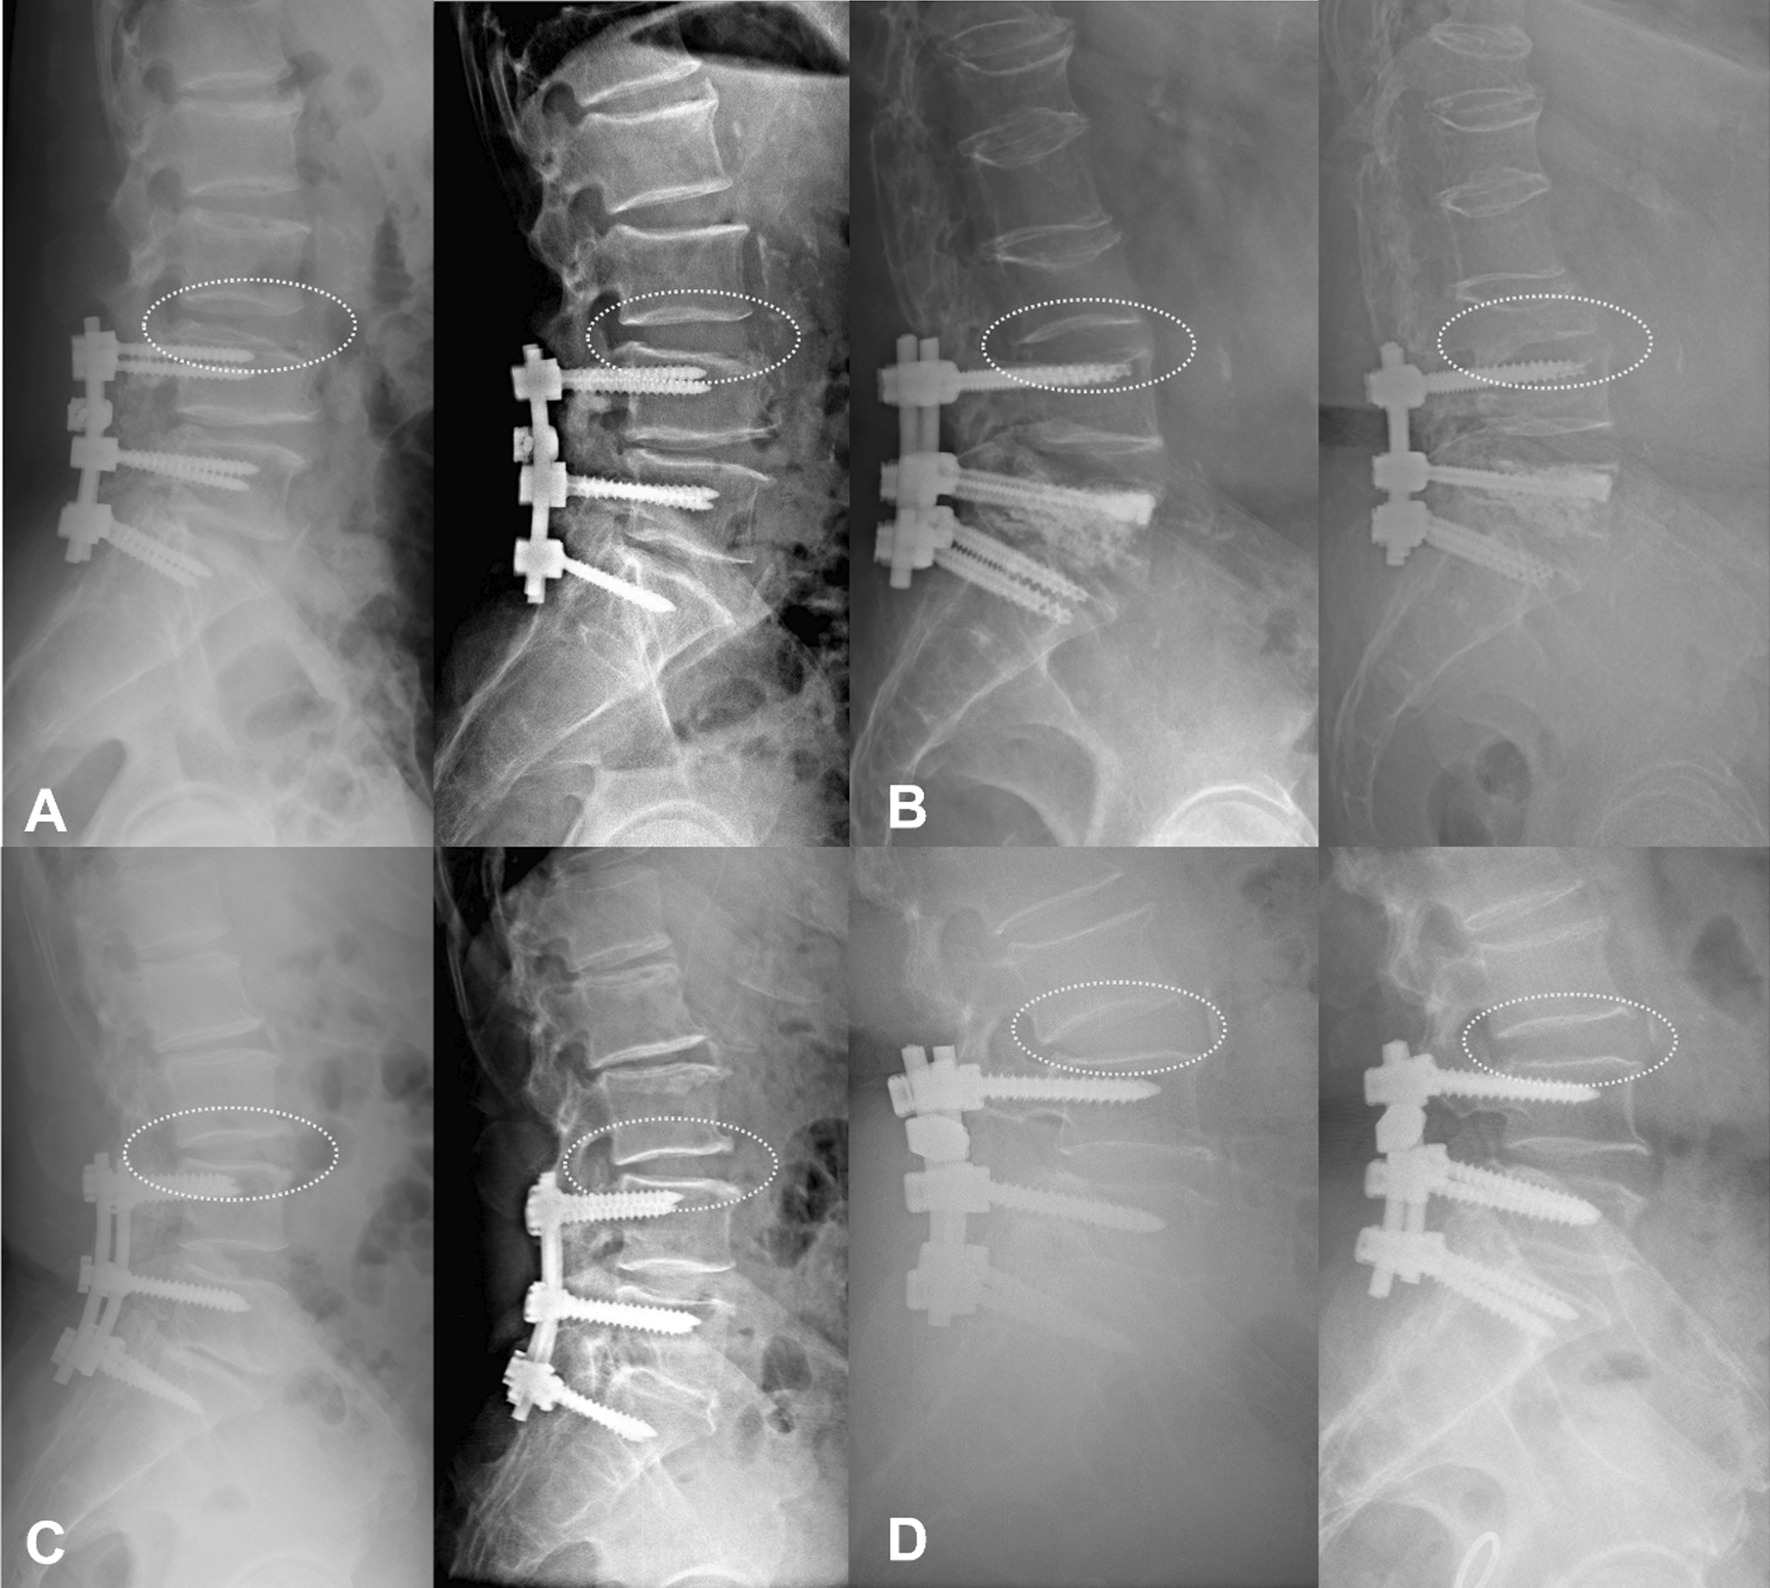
Comparison of ASD diagnosis-related parameters perioperatively and at the last follow-up by lateral radiograph. A non-ASD patient without pathological adjacent vertebral and intervertebral disk changes. B Narrowing of disk height (> 3 mm) accompanied by wedge compression of the upper vertebral body. C posterior slippage (> 3 mm) of L3 vertebral. D posterior opening at L3–4 in the flexion position after surgery (> 5°)
